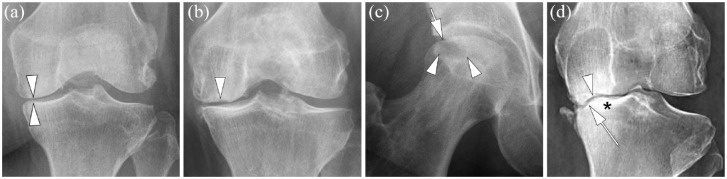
Examples of exclusionary radiographic findings at screening that result in non-eligibility in a-NGF/TrkA programs. (a) Atrophic osteoarthritis (OA). Atrophic OA is defined as a joint with definite joint space narrowing but no or only tiny osteophytes. Anteroposterior radiograph of a left knee shows definite joint space narrowing at the medial tibio-femoral compartment (arrowheads) without osteophyte formation classifying this knee as atrophic osteoarthritis. (b) Subchondral insufficiency fracture (SIF). Radiography is only able to depict the late sequelae of SIF, once definite osteochondral collapse of the joint surface has occurred. Joints with definite SIF at screening will not be eligible for inclusion. Anteroposterior radiograph of a left knee with definite medial joint space narrowing (i.e. Kellgren-Lawrence grade 3) shows medial femoral subchondral cortical depression as the result of SIF (arrowhead). In addition, there is surrounding sclerosis. (c) Osteonecrosis (ON). Early signs of ON are often equivocal on radiographs. Example shows definite ON with an area of subchondral radiolucency (arrowheads) and definite articular collapse (arrow) defining this joint as ON grade 3 according to the ARCO (Association Research Circulation Osseous) classification. (d) Rapid progressive OA Type II. Deformity of the medial femoral (arrowhead) and tibial articular surface (arrow) is noted in this anteroposterior right knee radiograph representing rapidly progressive osteoarthritis Type 2. In addition, there is articular surface collapse and marked subchondral sclerosis (asterisk), especially at the tibial plateau. Consequent varus deformity is observed.
